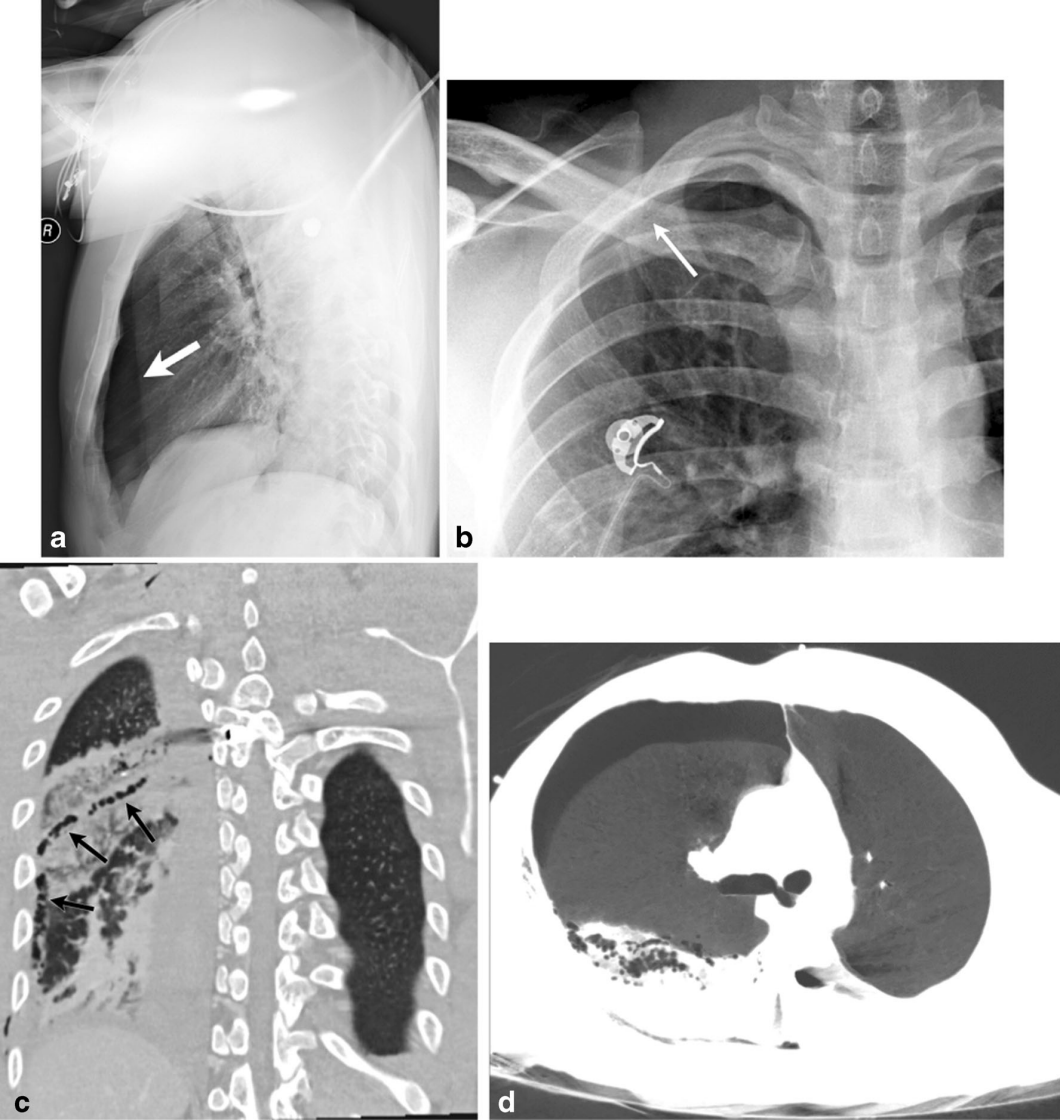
A 29-year-old male, status post gunshot wound. Emergently performed supine lateral chest radiograph at initial presentation (a) demonstrated an anterior right pneumothorax (*solid arrow*). Follow-up frontal chest radiograph (b) obtained 7 days later revealed a persistent right apical pneumothorax (*small white arrow*). Upon retrospective review, an alveolar- pleural fistula (*black arrows*) was present on this coronal reformatted image from the initial CT evaluation (c). This was secondary to lung laceration sustained along the bullet track. Axial minIP (d) image demonstrates the communication of this pulmonary laceration with the pneumothorax
